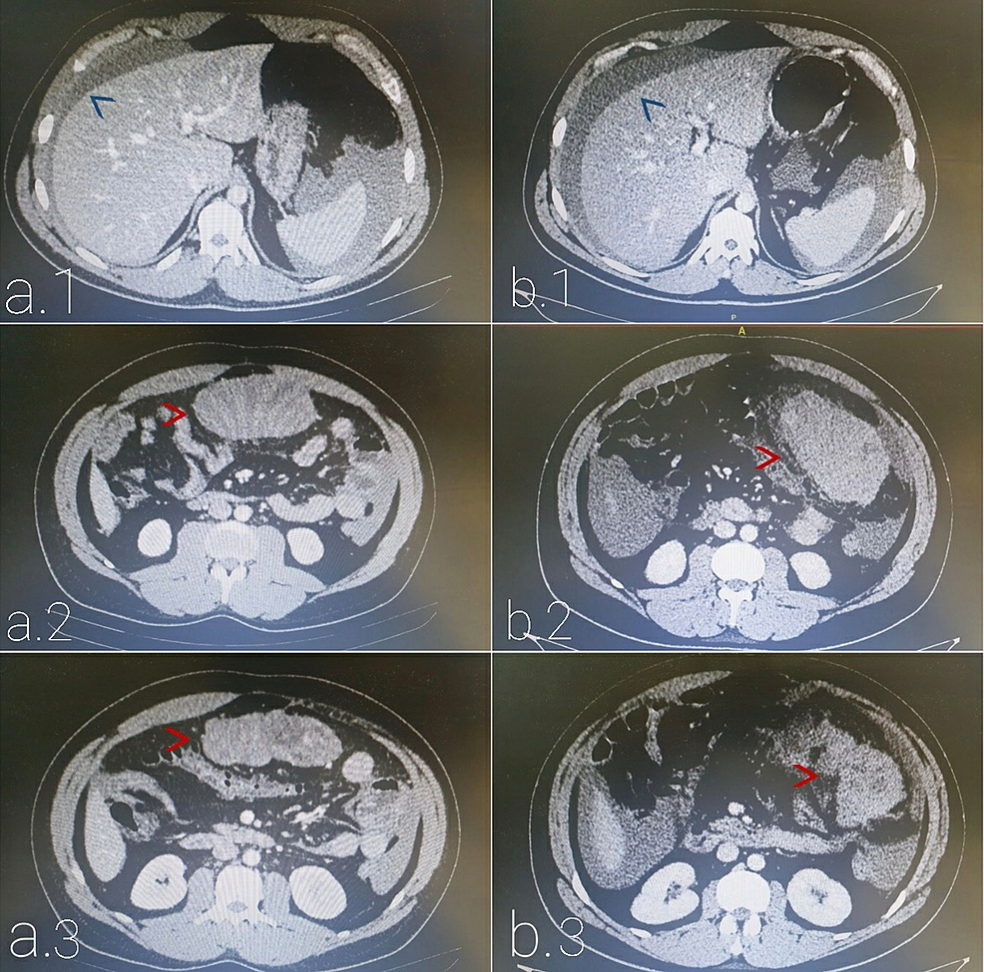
CT Scans of Abdomen and Pelvis a (1-3): CT scans with IV contrast done in the peripheral hospital. b (1-3): Urgent CT scans with IV contrast done at our institution. Blue arrow: perihepatic fluid; red arrow: gastric lesion
